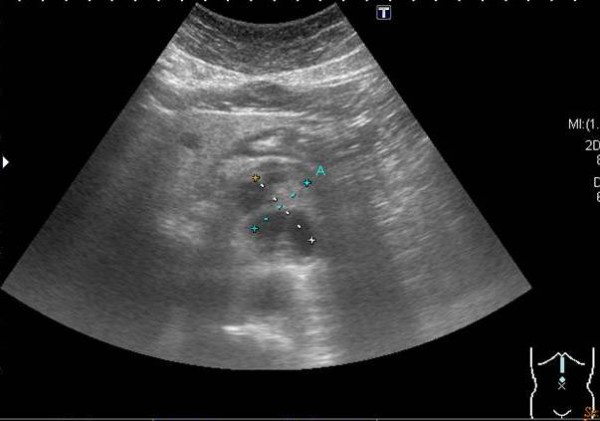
Ultrasonography findings. A mix-echoic lesion was near the pancreas.
